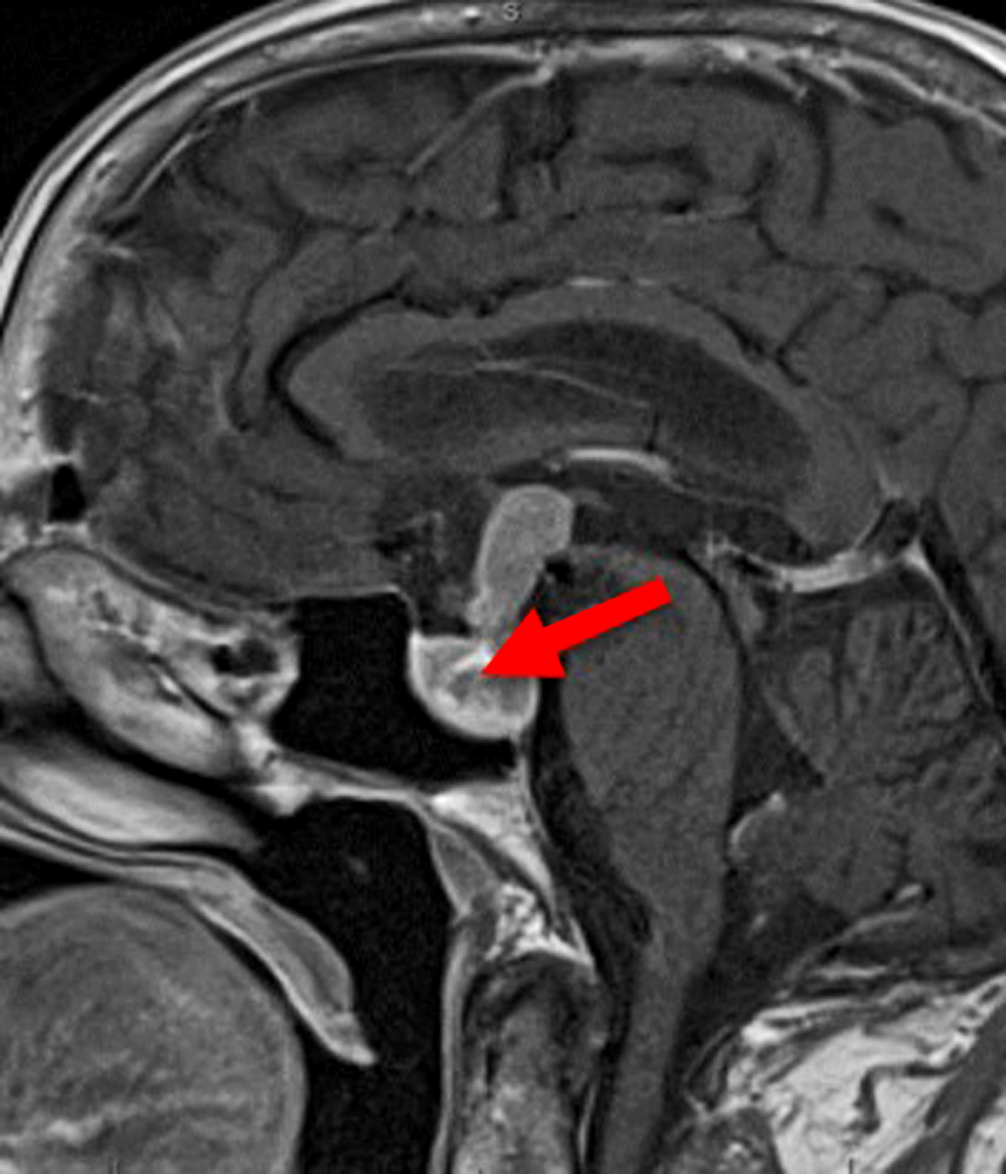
Sagittal view of the pituitary gland on a brain MRI. Arrow shows a 40 × 20.3 × 17 mm suprasellar pituitary macroadenoma associated with mild obstructive hydrocephalus.
